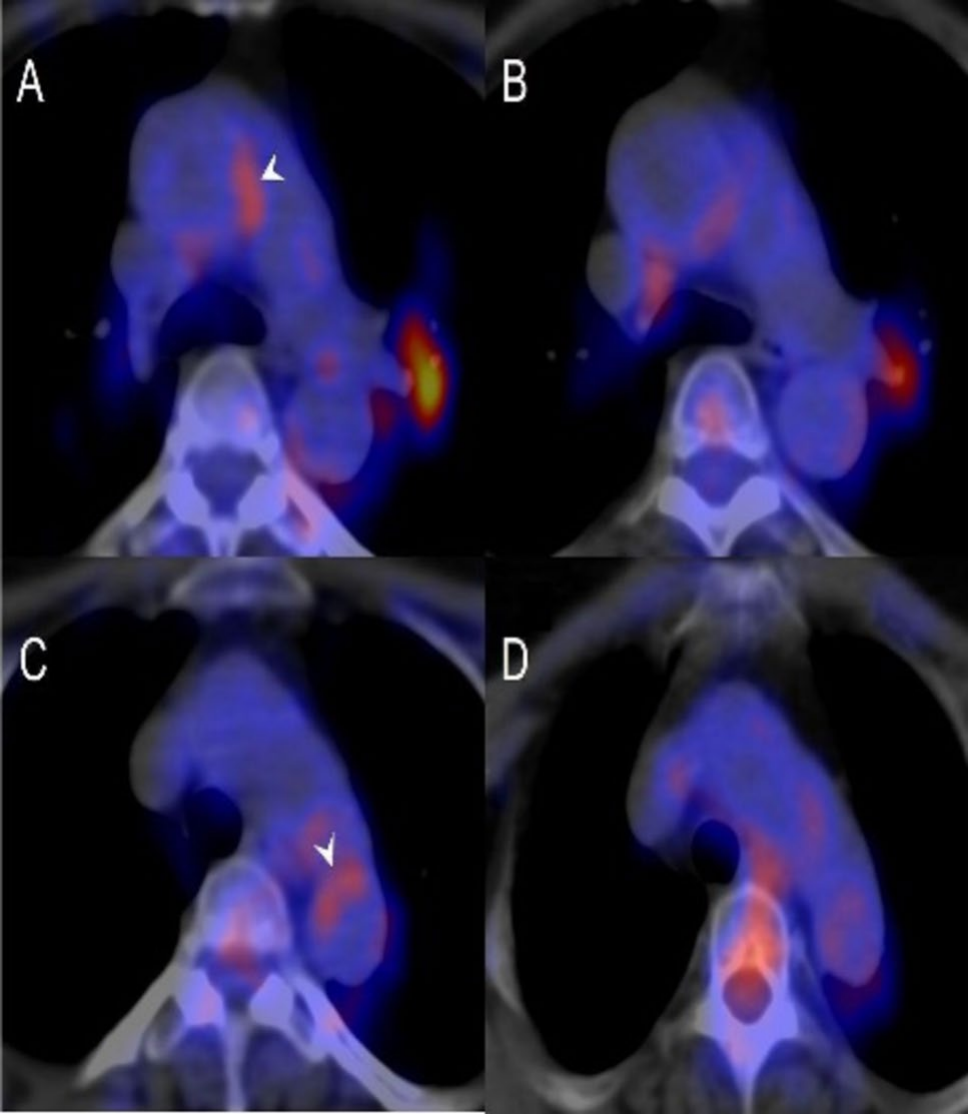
[^18^F]-FDG PET/CT images demonstrating aortic inflammation in patients with RA at baseline (A, C) and after therapy (B, D) with tofacitinib. With permission from reference [60]
